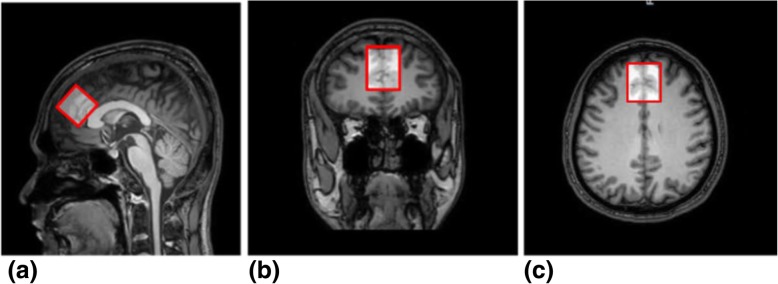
Position of MRS region of interest. A region of interest (ROI) with a size of 30 × 30 × 30 mm^3^ was created over the anterior cingulate cortex according to a high-resolution anatomic 3D T1WI MRI scan. The ROI is shown in (a) sagittal, (b) coronal, and (c) transversal orientations
